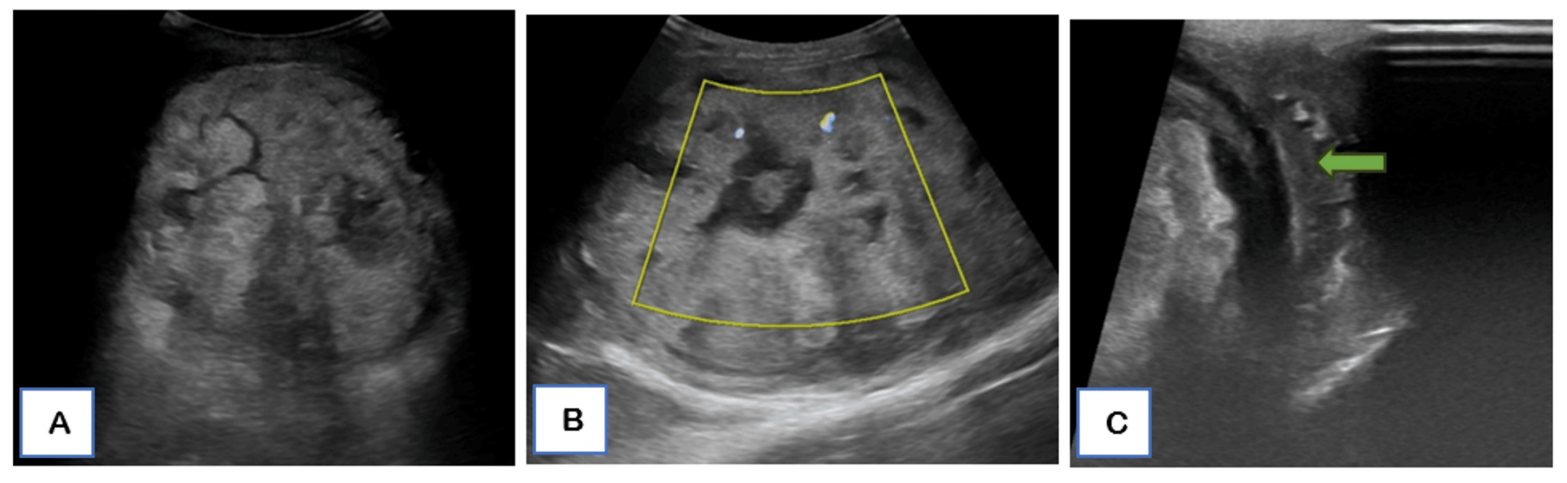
Scrotal ultrasound images (A) A well-defined, heterogeneous, predominantly hyperechoic lesion was seen in the left scrotum. (B) On color Doppler, it showed minimal vascularity. (C) The left testis was visualized separately; however, it was significantly compressed (green arrow).
